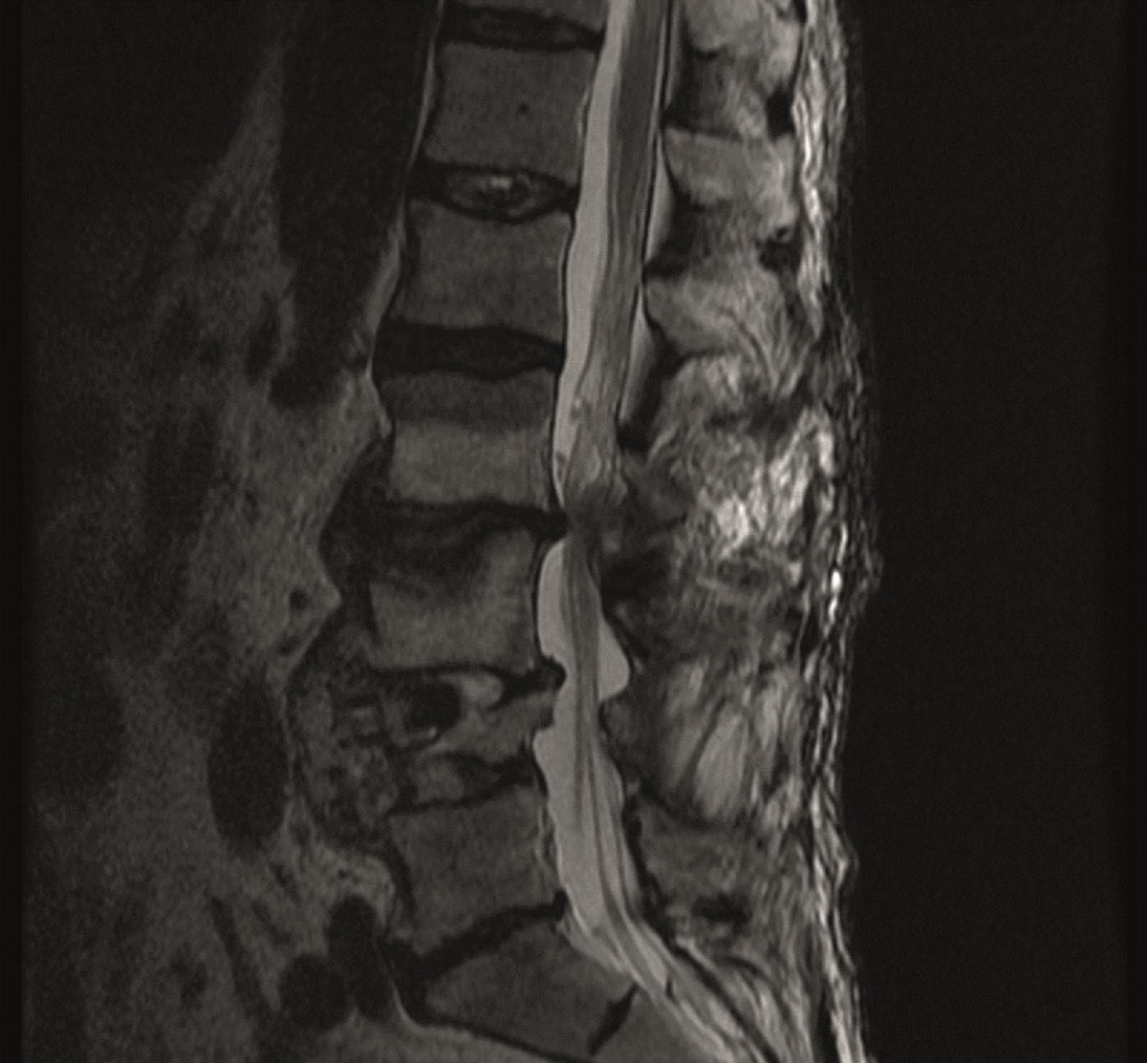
Sagittal T2 Pre-operative MRI
